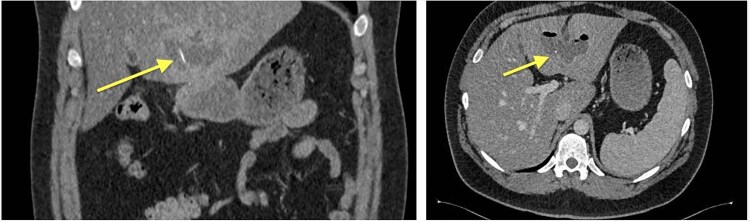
Abdominal CT, hyperdense foreign body (metallic wire).
